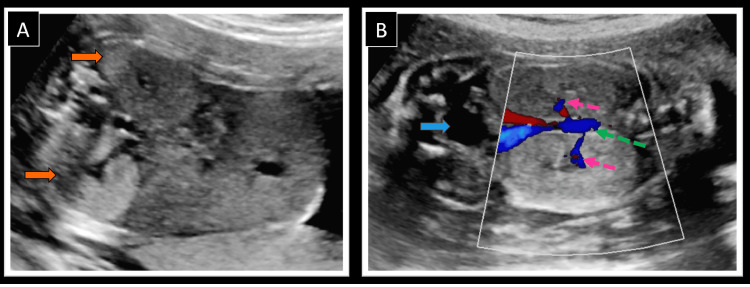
Fetal abdominal ultrasound showing bilateral enlarged dysplastic kidneys (A) Transverse ultrasound image through the fetal abdomen showing symmetrically enlarged and echogenic bilateral kidneys (orange arrows). (B) Coronal color Doppler ultrasound image showing abdominal aorta (green arrow) giving off two normal caliber renal arteries (pink arrows) entering renal hilum. A partially distended urinary bladder is also seen in the image (blue arrow).
